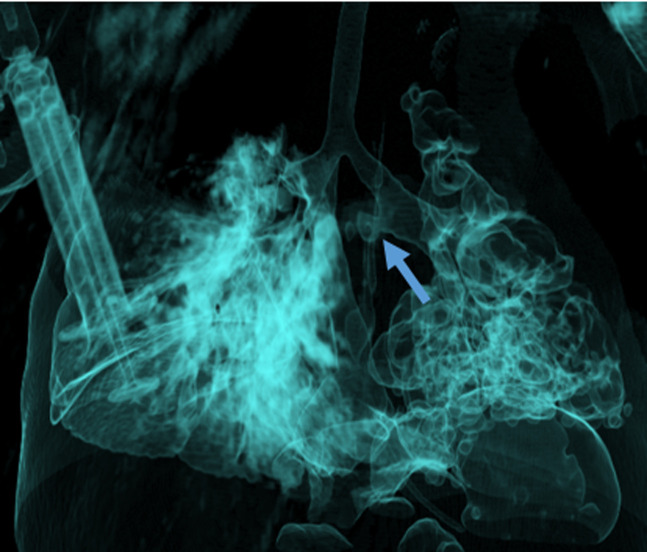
a volume-rendered computed tomography image showing fistulous connections between the distal left main bronchus and the esophagus
